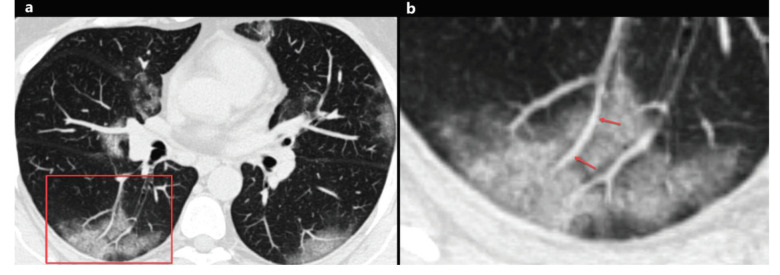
A 46-year-old woman with COVID-19. Axial chest CT image shows peripheral and peribronchovascular distributed focal ground-glass opacities in both lungs, and focal vascular enlargement is seen inside the ground-glass opacity in the right lower lobe (a, red frame). The magnified image of the red frame shows vascular enlargement inside the ground-glass opacity (b, arrows).
